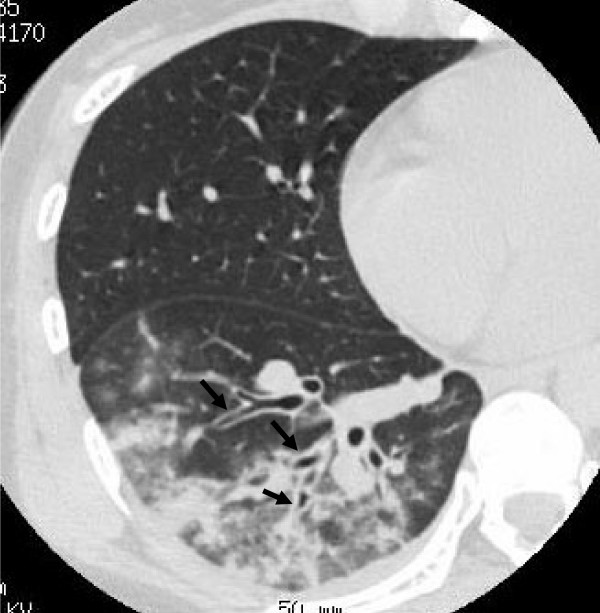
30-year-old woman with *M. pneumoniae *pneumonia. CT shows bronchial wall thickening (arrows). Lobular areas of consolidation and ground-glass attenuation are also seen.
